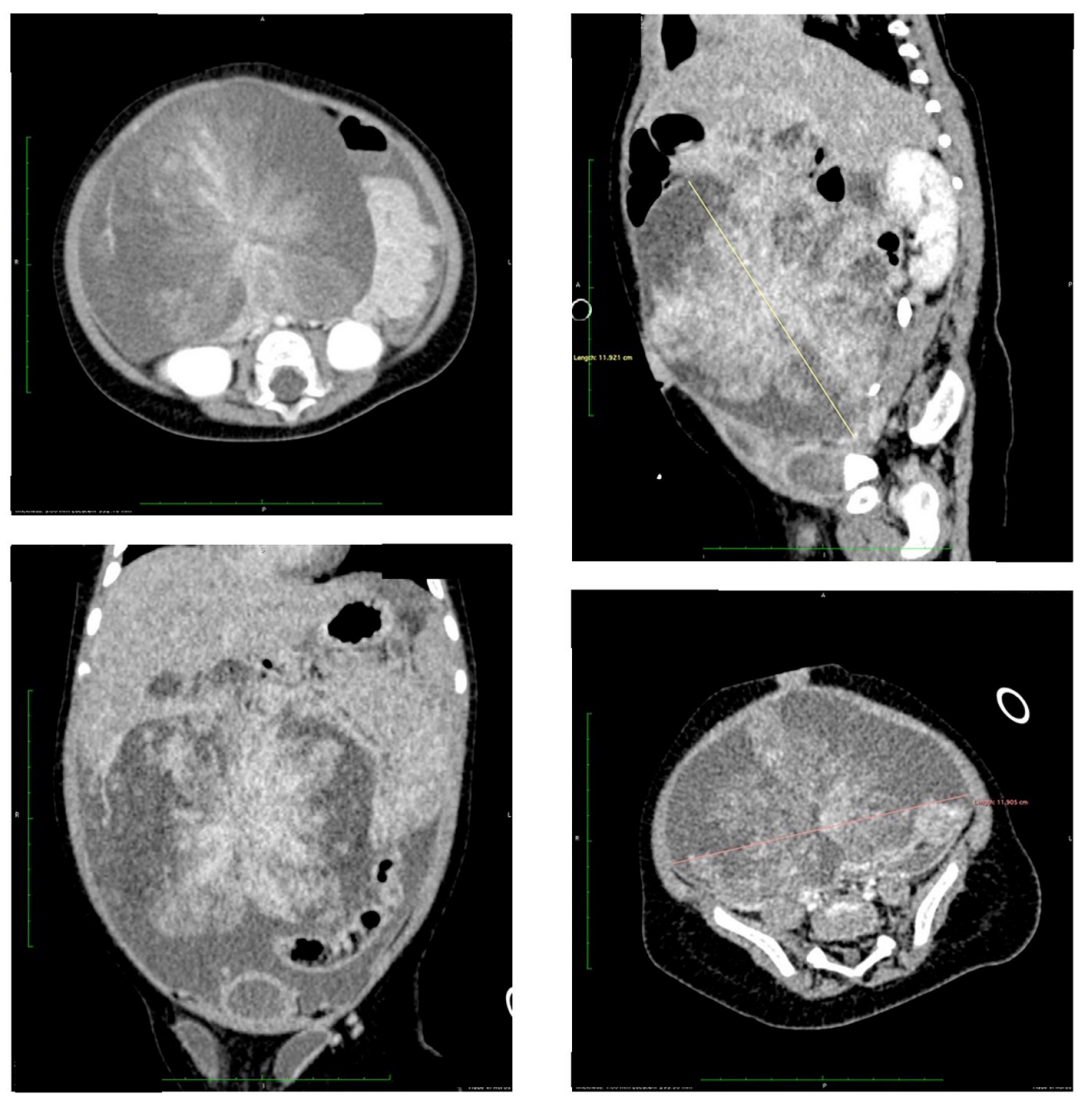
Pre-operative CT scan: a solid-cystic mass (113 × 98 × 103 mm) in the right abdominal area.
